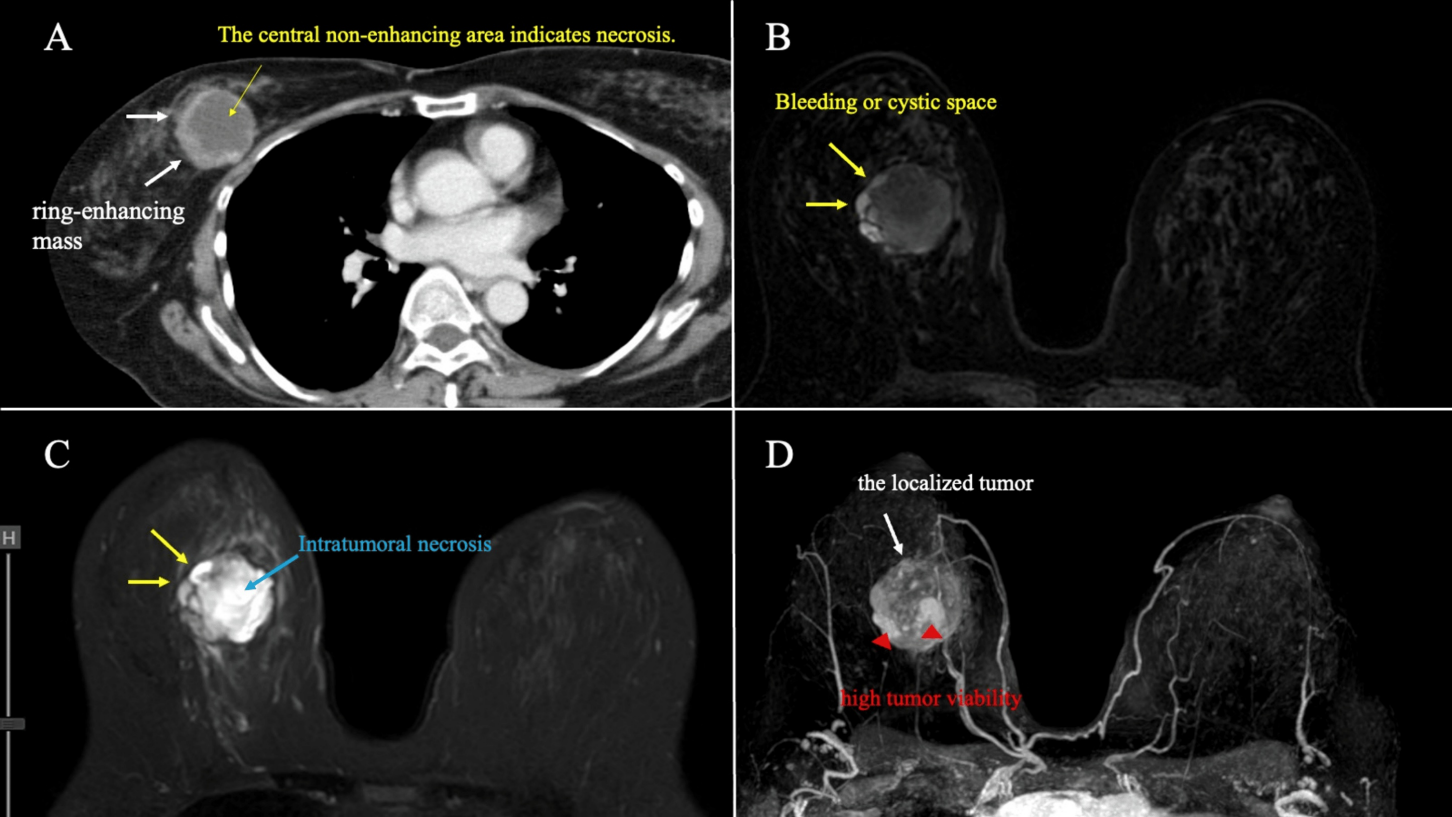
Contrast-enhanced CT and MRI findings. (A) CT showed a ring-enhancing mass (white arrow). (B, C) The tumor margins exhibited elevated SI on both T1WI and T2WI in MRI (yellow arrow). The yellow arrows indicating internal bleeding or cystic regions. (D) MIP images show the localized tumor (white arrow) with no obvious intraductal spread. Tumor showed areas of high proliferative potential (red arrowheads). CT: Computed tomography, MRI: Magnetic resonance imaging, SI: signal intensity, WI: weighted images, MIP: Maximum intensity projection.
